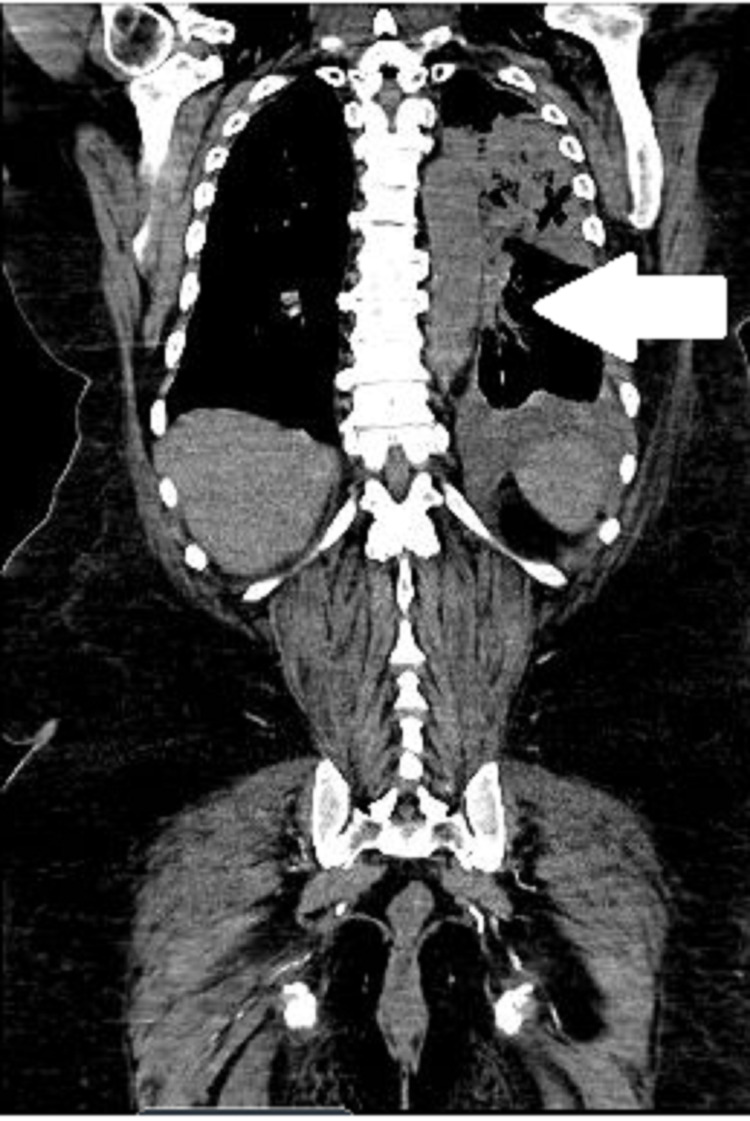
CT chest and thorax showing multiple enlarged mediastinal lymph nodes measuring up to 1.3 cm. Other left hilar and lower mediastinal lymph nodes are also noted to be enlarged.
